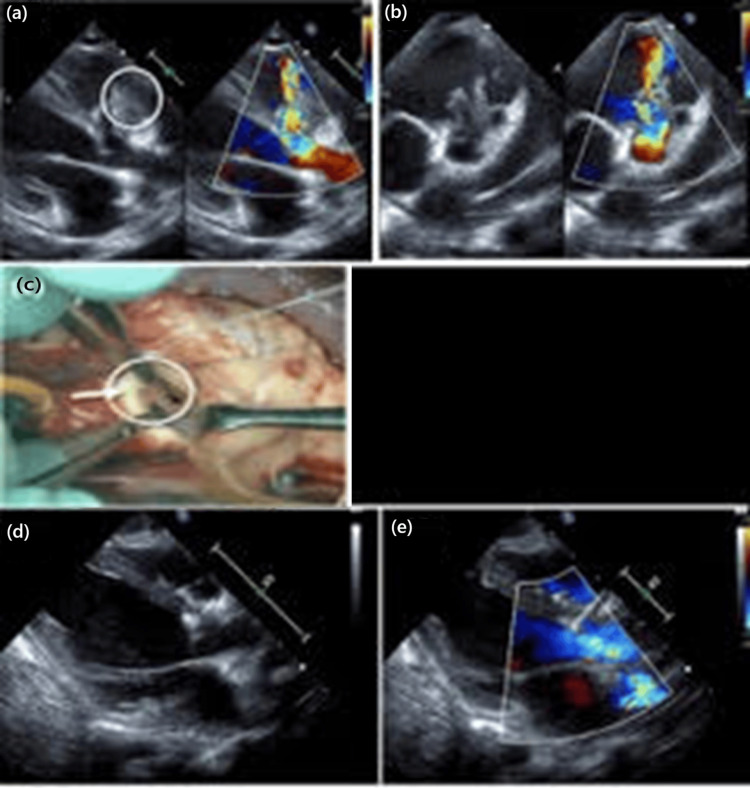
Serial echocardiogram and surgical findings. (a) Transthoracic echocardiogram (TTE) on the 11th day after admission in the long-axis view before surgery. TTE shows a large vegetation (white circle) above the aortic valve and sinus of Valsalva aneurysm rupture with right coronary cusp prolapse and left-to-right (L-R) shunt. (b) TTE on the 11th day after admission in the short-axis view with L-R shunt. (c) The surgical findings show severe destruction of the right cusp (white arrow) and a perforated aortic sinus of Valsalva (white circle). (d) TTE after surgery in the long-axis view. (e) TTE after surgery in the long-axis view, with closure of the rupture and diminished L-R shunt with trivial aortic regurgitation.
